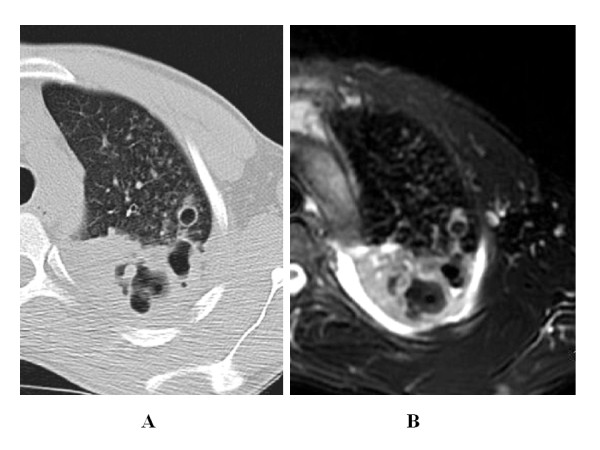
32 year-old man with pulmonary tuberculosis. A) HRCT shows parenchymal consolidation with cavitations. B) Unenhanced MRI better depicts caseosis, air level in the cavities and pleural effusion.
